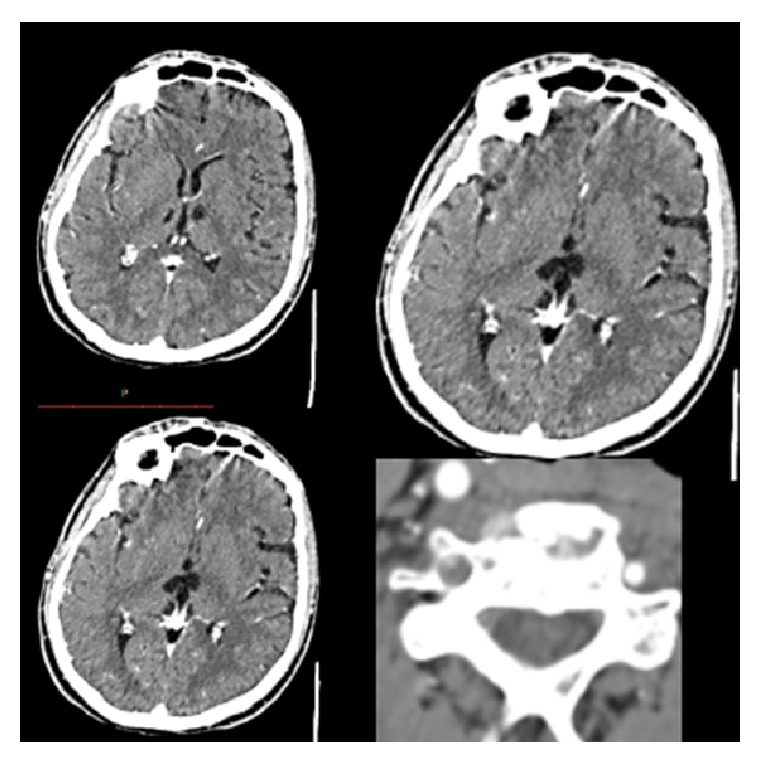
Brain and cervical CT Angiography (after 3 months) revealed a bilateral hypodense area in the site of both thalami with no recanalization of right vertebral artery.
